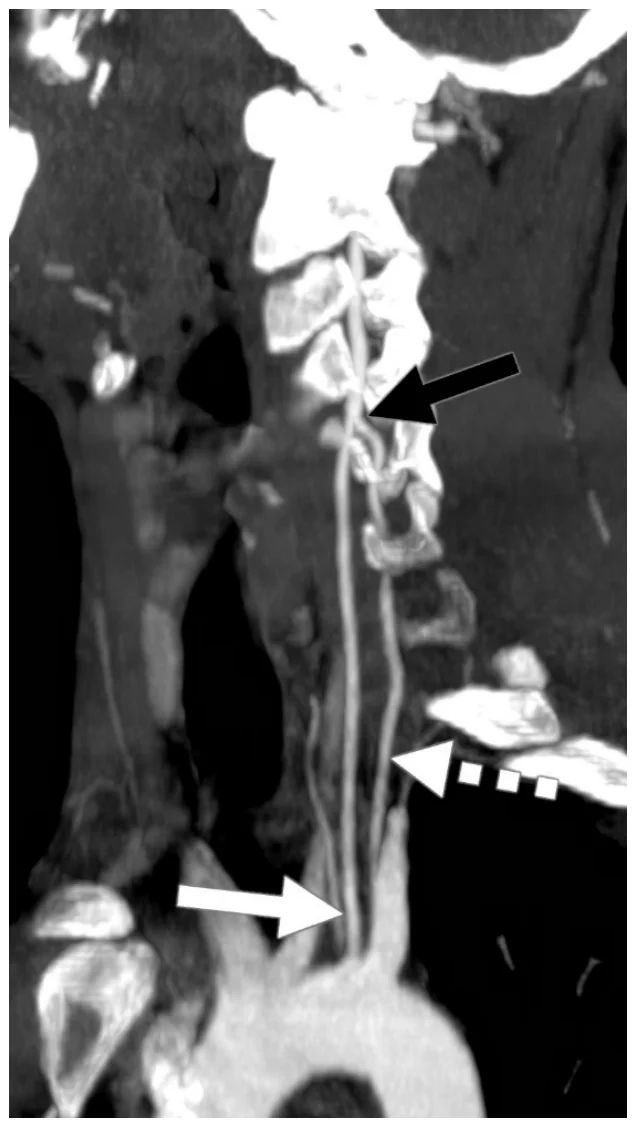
Dual origin of the left VA. Oblique sagittal CTA MIP shows the dual origin of the left VA from the aortic arch (white arrow) and the left subclavian artery (dashed white arrow), merging at the C4–C5 level (black arrow). The aortic arch branch enters the transverse foramen after the confluence.
